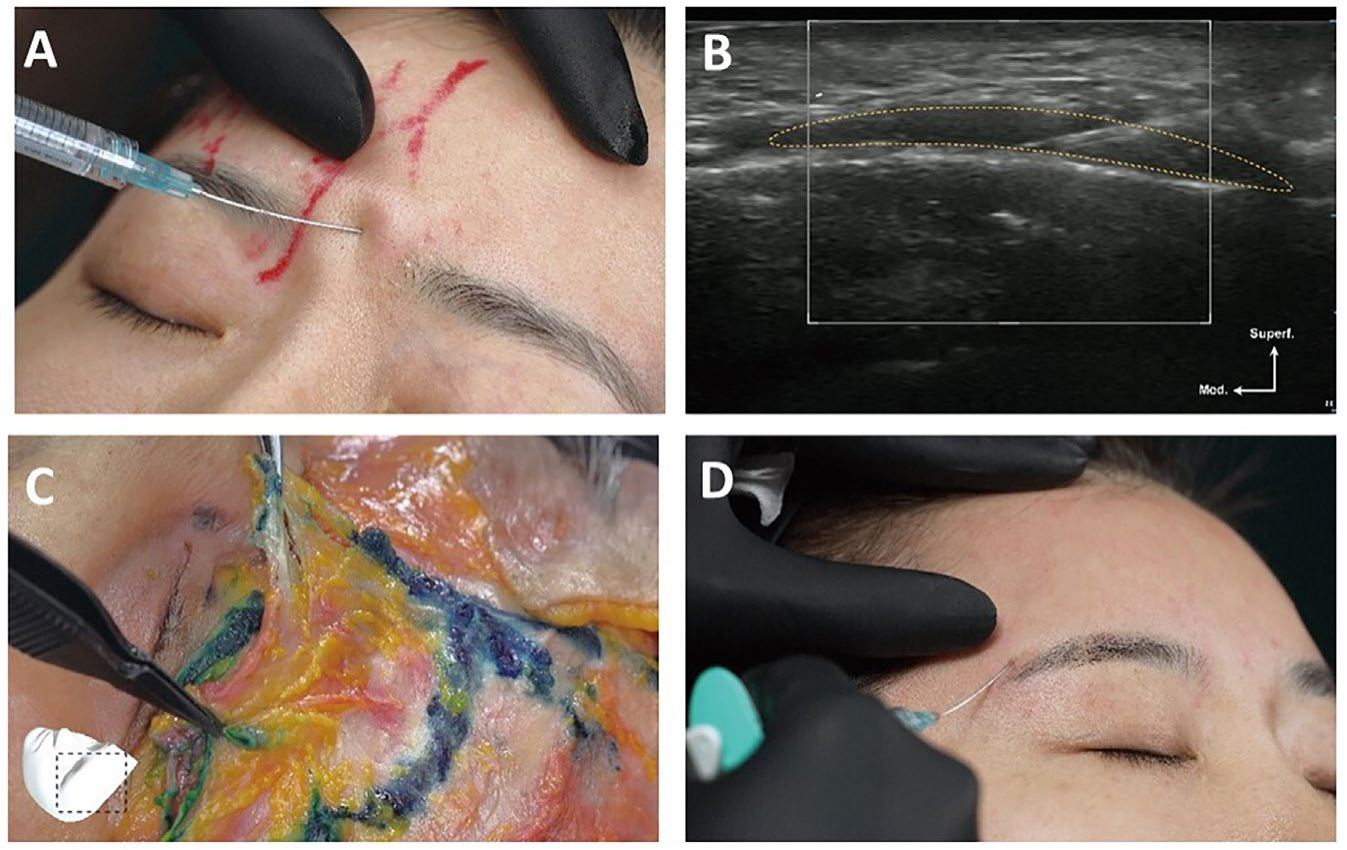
A demonstration of the midpoint approach for the forehead (41‐year‐old Korean female). (A) Clinical injection. (B) Clinical ultrasound. (C) Cadaver dissection (72‐year‐old Korean male). (D) A photo of the lateral approach for the forehead.
